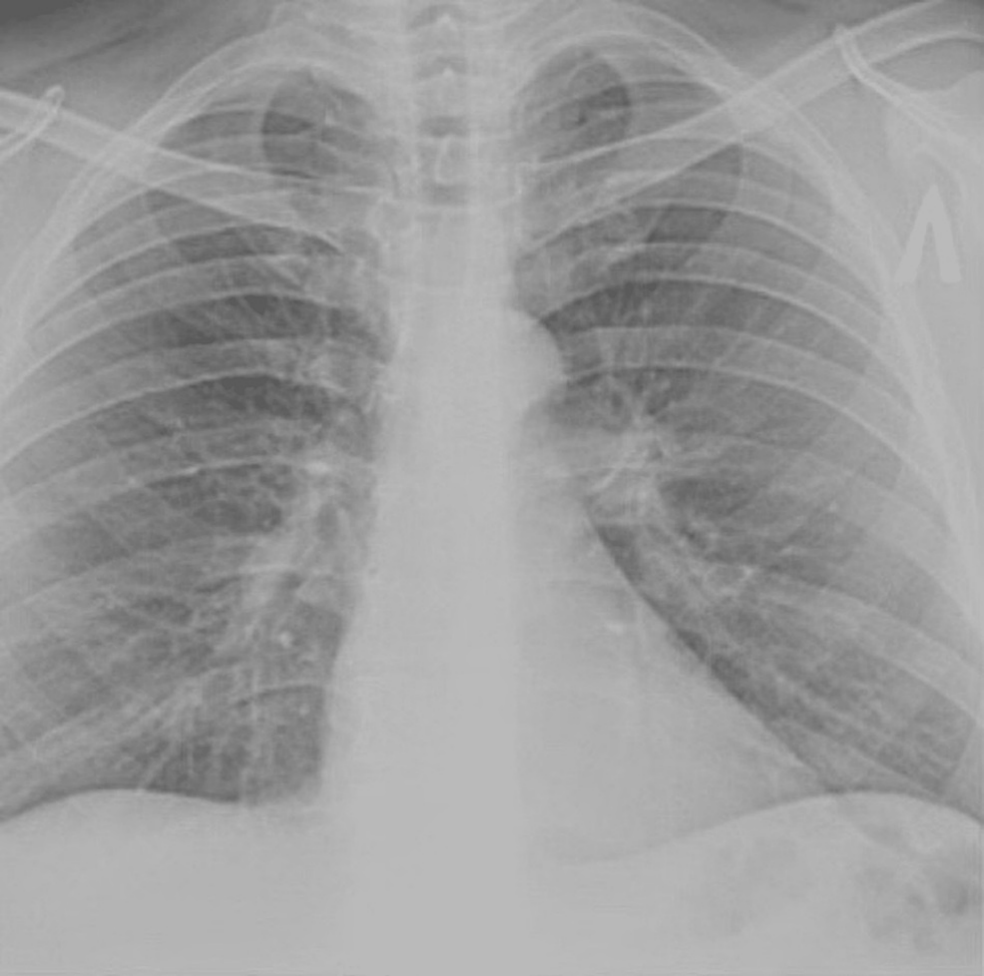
TB chest X-ray dataset example TB, tuberculosis.
